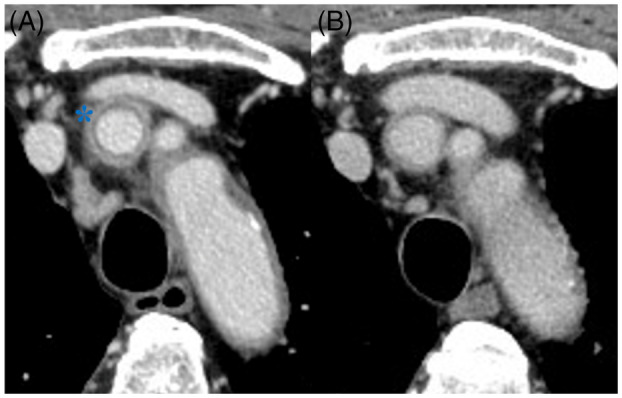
Venous phase of enhanced computed tomography (CT) findings. (A) CT performed 8 days after the administration of filgrastim revealed thickening of the brachiocephalic artery wall and enhancement with a double‐ring sign (asterisk). (B) CT performed after the administration of prednisolone demonstrated decreased arterial wall thickening without the double‐ring sign.
